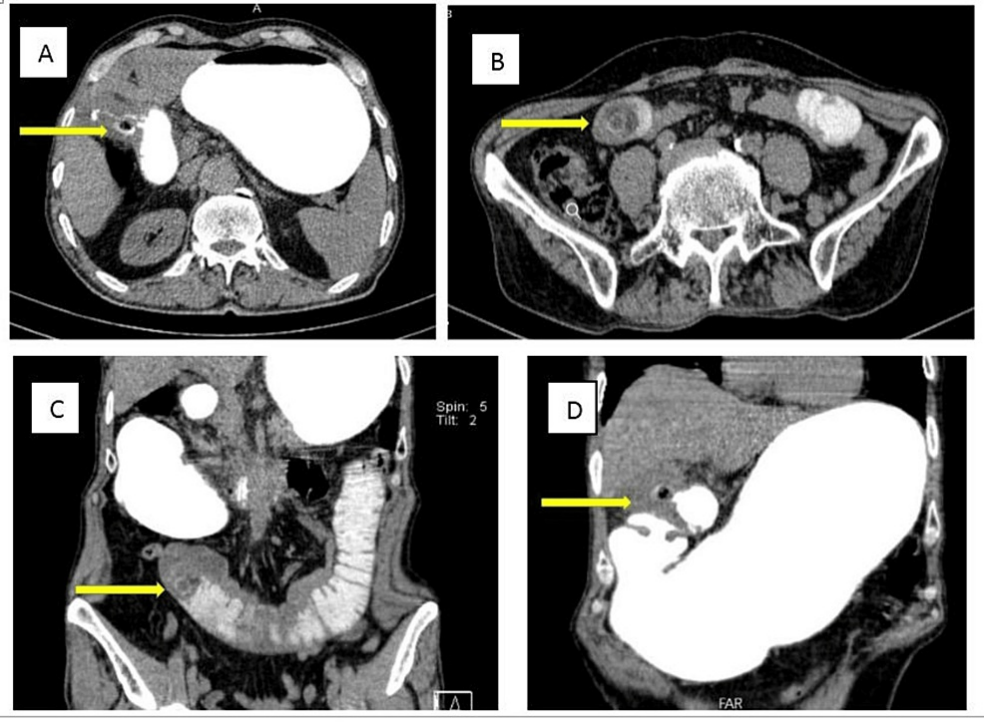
Oral contrast-enhanced CT - 1 The images demonstrated dilated jejunal loops (B, C), fistulous tract between gallbladder wall and duodenal bulb (A, D), oral contrast leakage to gallbladder lumen (A), obstructing layering gall bladder stone in the jejunum (B, C) compatible with gallstone ileus CT: computed tomography
